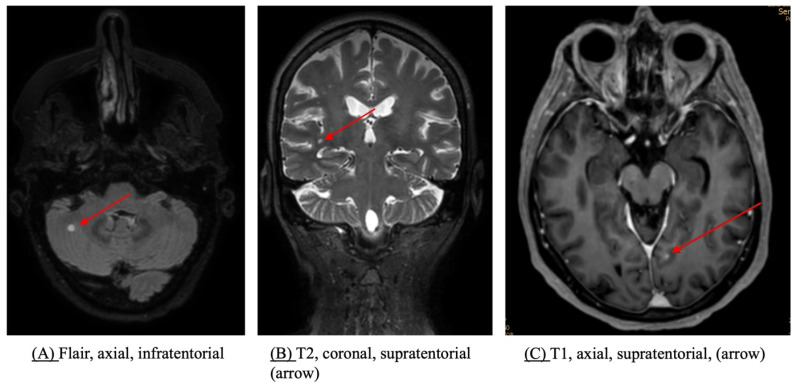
MRI in the detection of BMs. Red arrows indicate the identified BM in different MRI modalities and locations with an (A) infratentorial metastasis in flair, (B) a supratentorial metastasis in the T2-sequence, and (C) a paramedian metastasis in the T1-sequence. Abbreviations: BM = brain metastases, MRI = magnetic resonance imaging.
